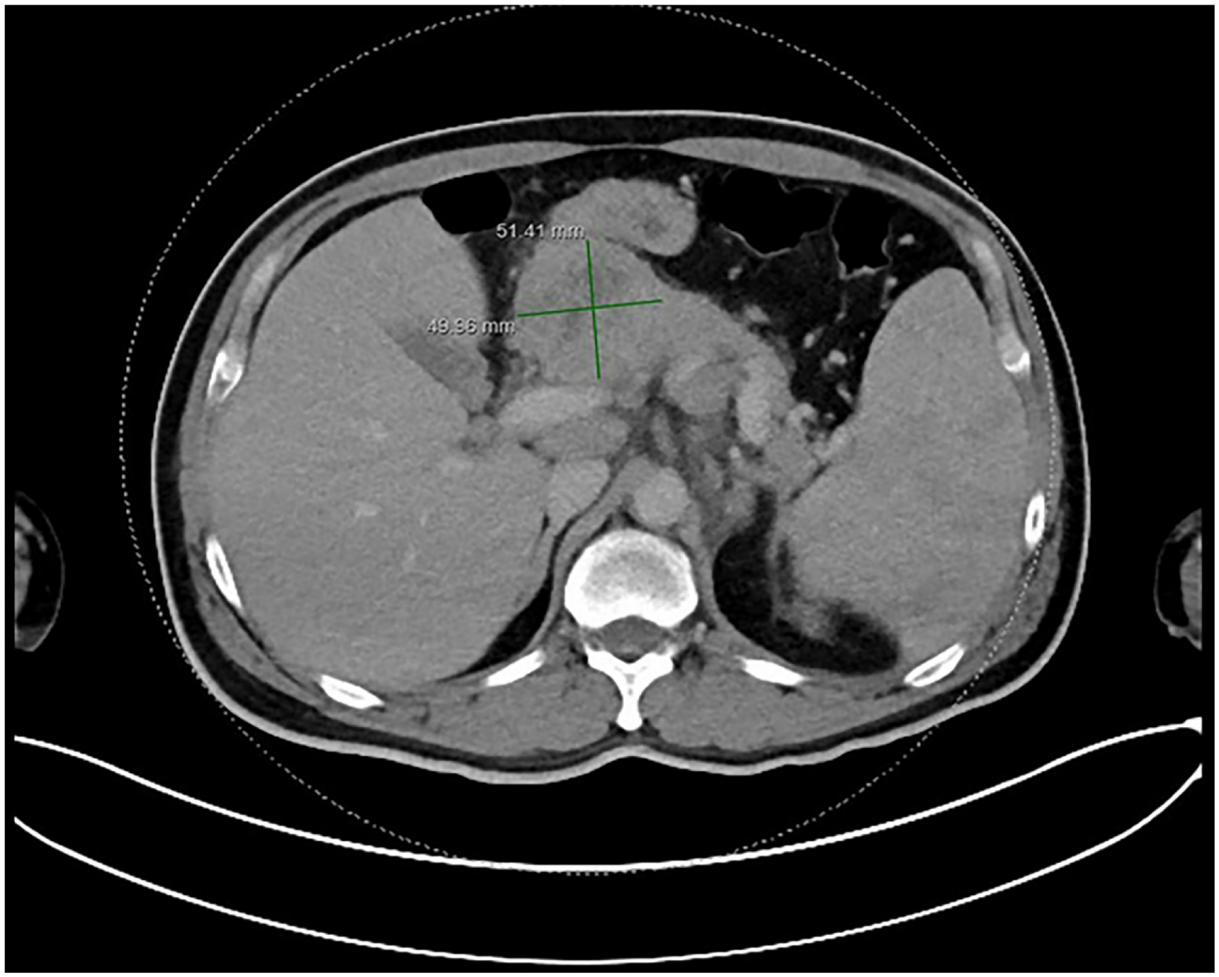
CT scan Abdomen axial view showing lesion within pancreas/adjacent peripancreatic lymphadenopathy.
